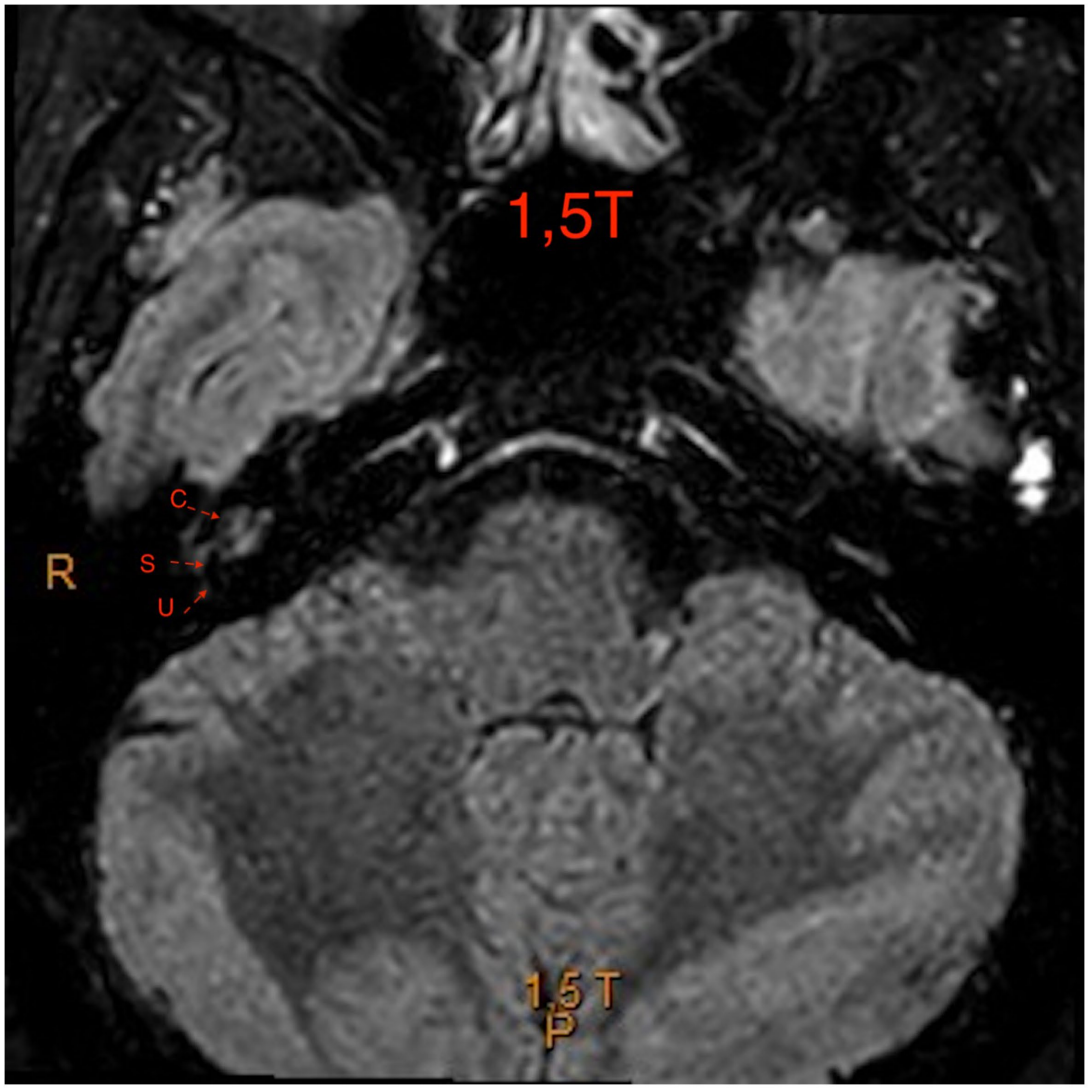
1.5 T MRI axial scan, sequence 3D-FLAIR, right ear with grade 2 of Bárath in cochlea and vestibule.
